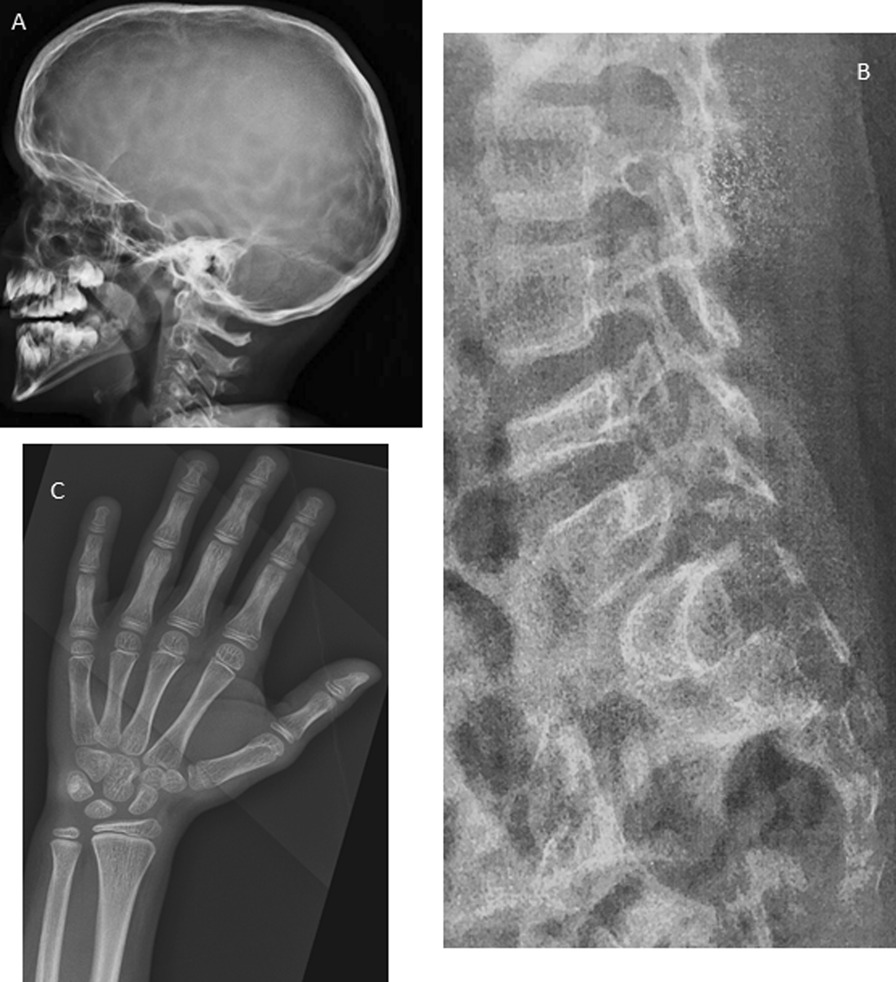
Radiological features. A. Skull X Ray (9 years 3 months girl). Note the thin cranial vault, the demineralization and the digitiform Impressions. B. Spine X Ray (10 years boy). Note the demineralization, the vertebral fracture, and the thin cortical bone. C. Hand X-Ray (9 years 3 months girl). Note the demineralization, the delayed bone age (age 6 years 10 months), small epiphyse, and striated metaphyses
